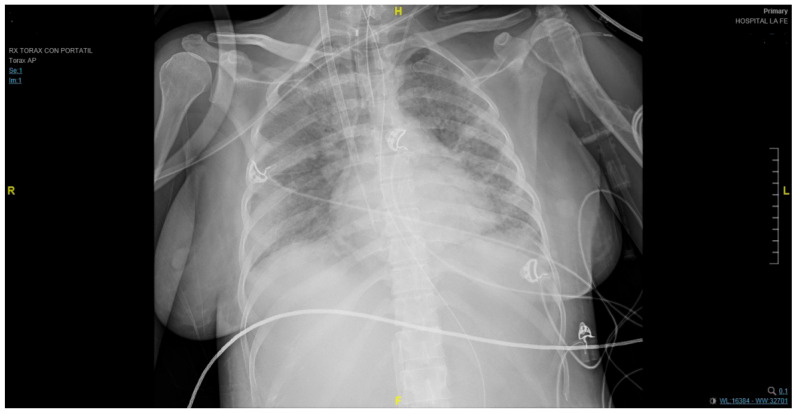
An anteroposterior chest X-ray revealed extensive bilateral areas of consolidation and the endotracheal tube at 2 cm of carina.
